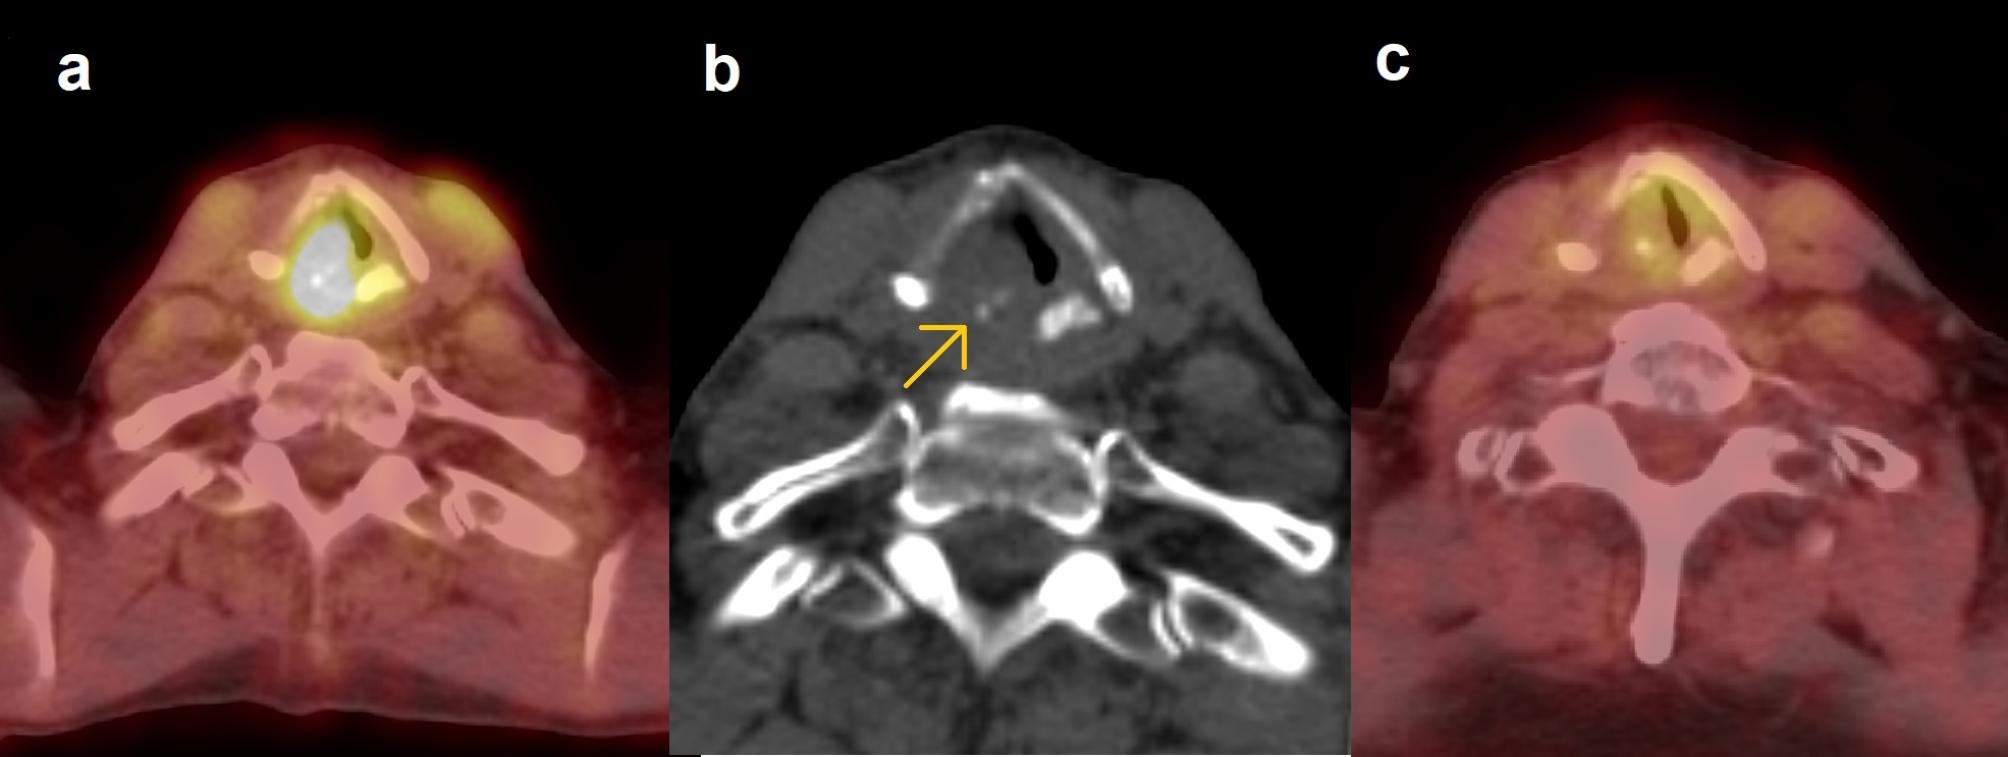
Example of a false positive interpretation in the case of cricoid cartilage radionecrosis in a T4aN0 tumor. (a) Post-treatment PET-CT showed a markedly increased uptake of SUV_max_ 8.3 at the right posterior vocal cord and arytenoid region, along with a mass, at the site of the primary tumor. The axial image (b) shows small, fragmented arytenoid cartilage (arrow). A subsequent laryngoscopy revealed defects in the mucosa and exposed cartilage. The biopsy was negative for residual disease. The patient developed difficulty in breathing and a laryngeal oedema, and was tracheostomized. Symptoms resolved after antibiotics and hyperbaric oxygen therapy, which were started due to suspected chondroradionecrosis. (c) In the follow-up PET-CT 8 months later, SUV_max_ had markedly decreased to 3.6
